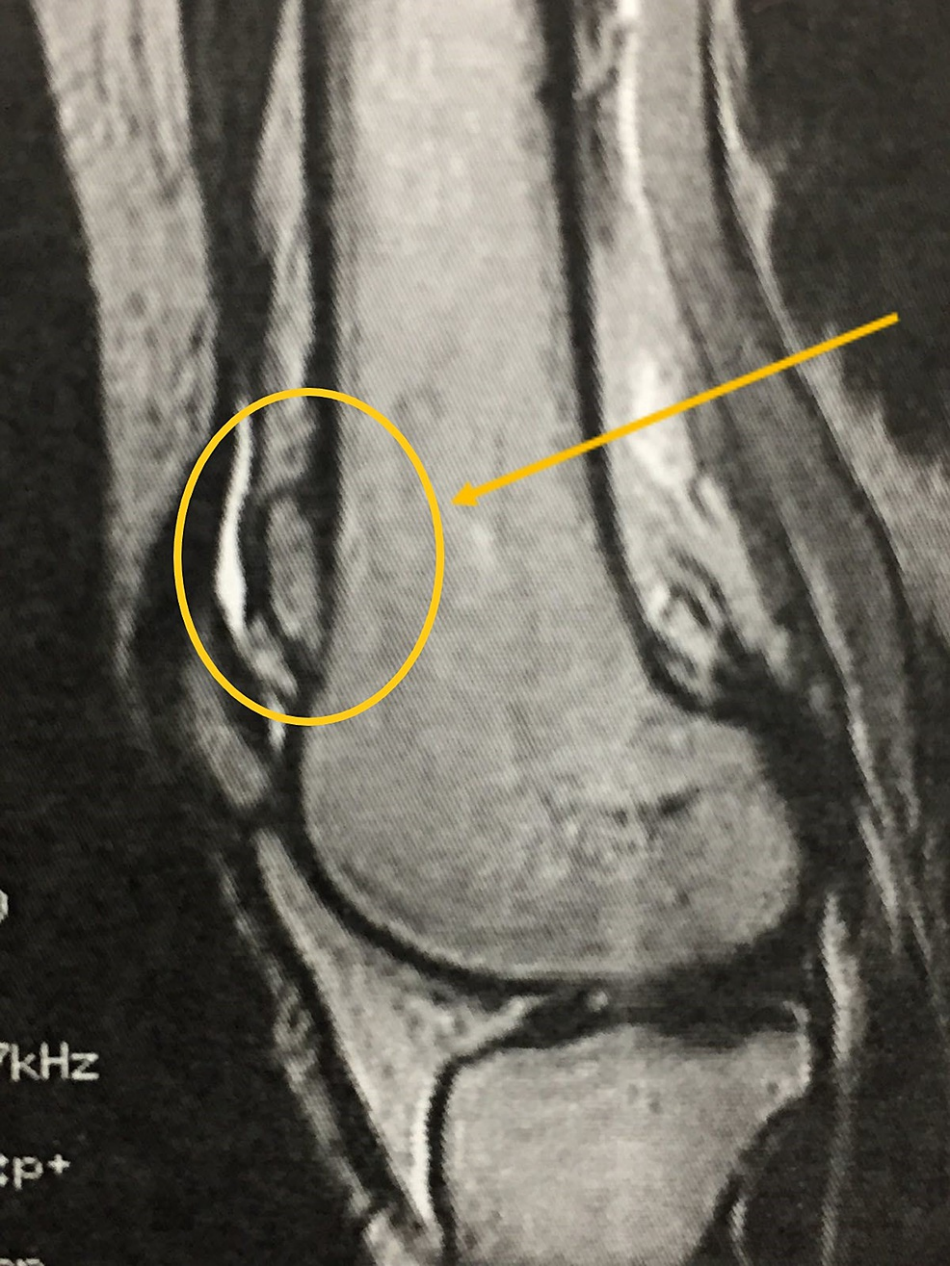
Enhanced image of the MRI sagittal plane where the bone lesion can be seen arising from the femoral bone (yellow circle and arrow).
